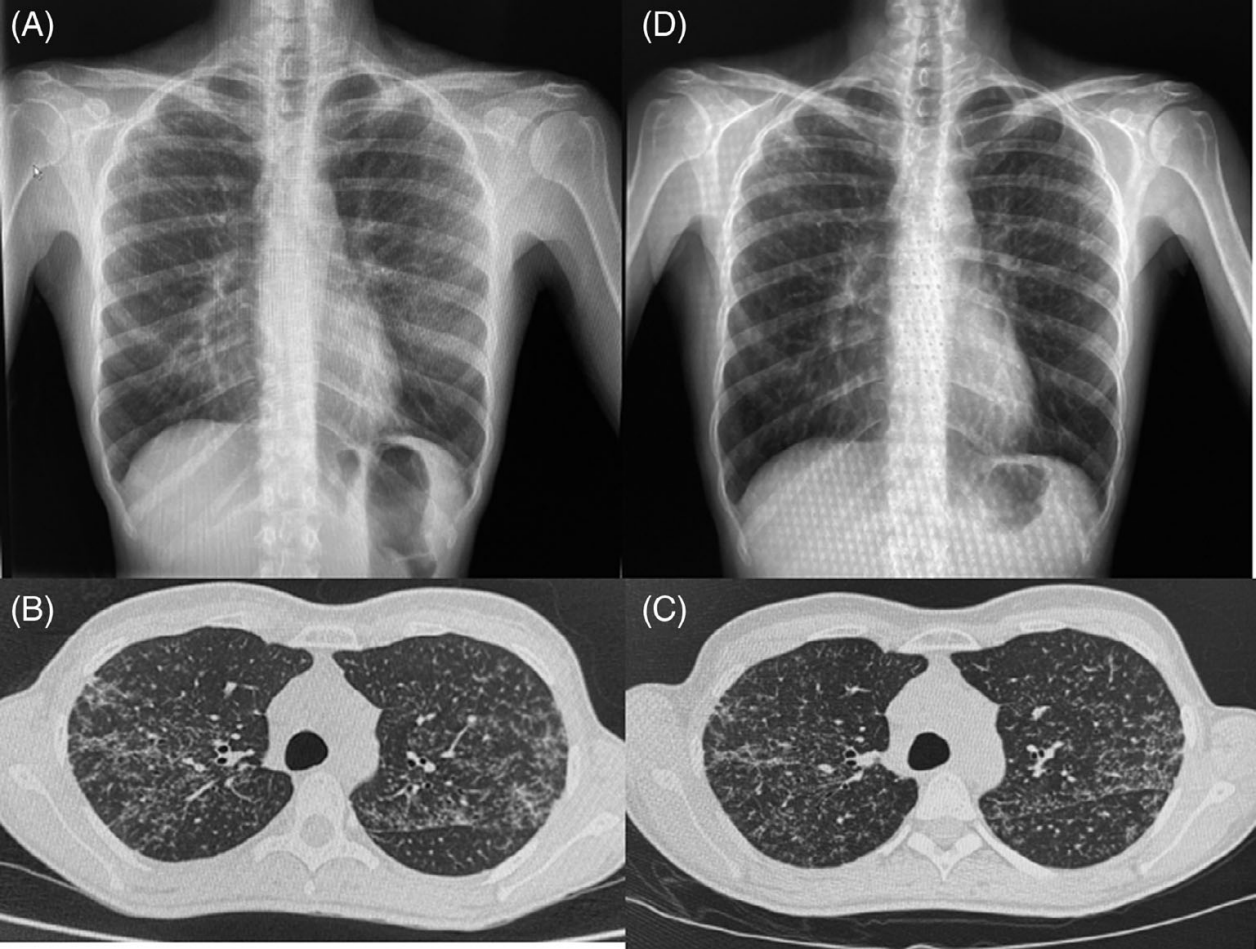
(A) Chest x‐ray performed in March 2020 showing discrete diffusely distributed and bilateral pulmonary infiltrates. (b) Chest computed tomography (CT) performed in May 2020 objectifying diffuse and bilateral micronodular pulmonary infiltrates, predominantly in the upper lobes. (C) Chest CT performed in November 2020 showing no radiological changes. (D) Chest x‐ray performed in June 2021 showing stability of pulmonary infiltrates
